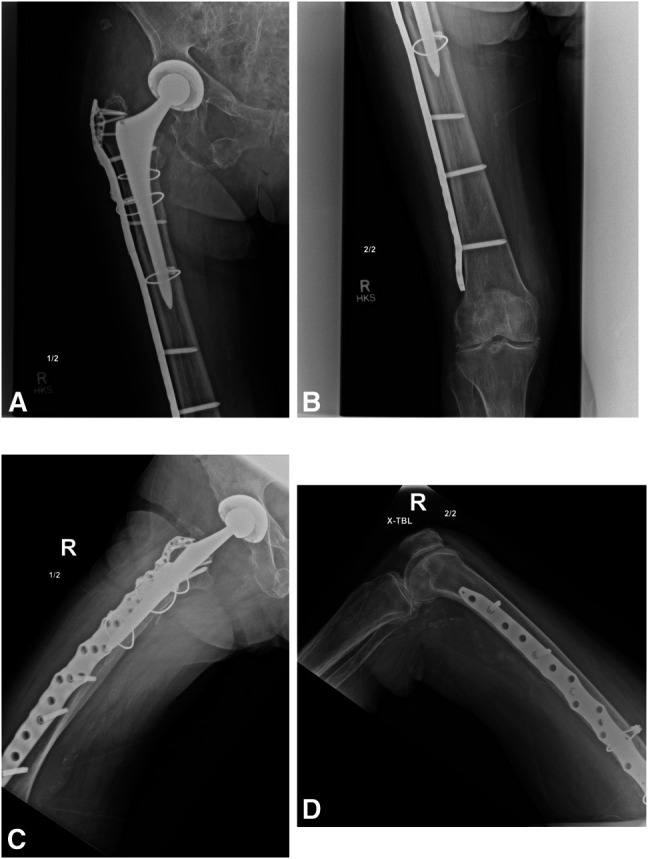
A and B, AP and (C and D) lateral radiographs of the right femur obtained 7 weeks postoperatively demonstrate appropriate fracture healing and no evidence of implant failure.
